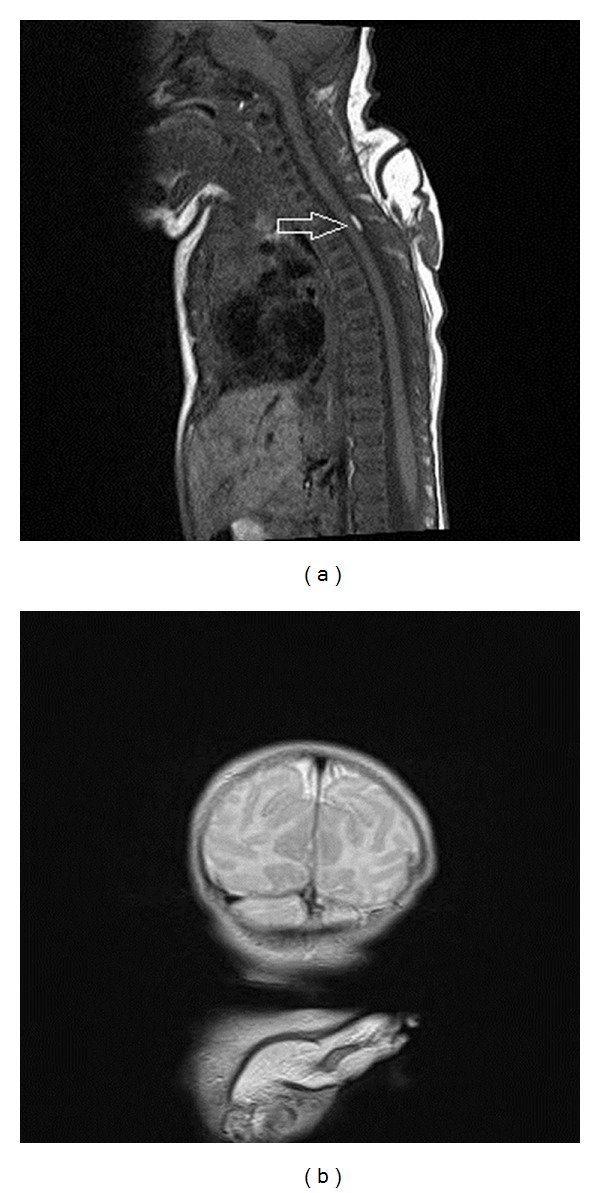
T1-weighted sagittal (a) and T2-weighted coronal (b) images. Arrow points that the lipoma is located at the same level of the ectopic extremity. Medulla Spinalis terminates between L3 and L4 vertebrae. Ectopic extremity originates from subcutaneous soft tissues and it does not extend deeper neural elements. It ends interspinous ligament. Coronal T2-weighted image shows dorsally located extremity, composed of fat and muscle tissue. Note that cerebral structures are in normal limits.
